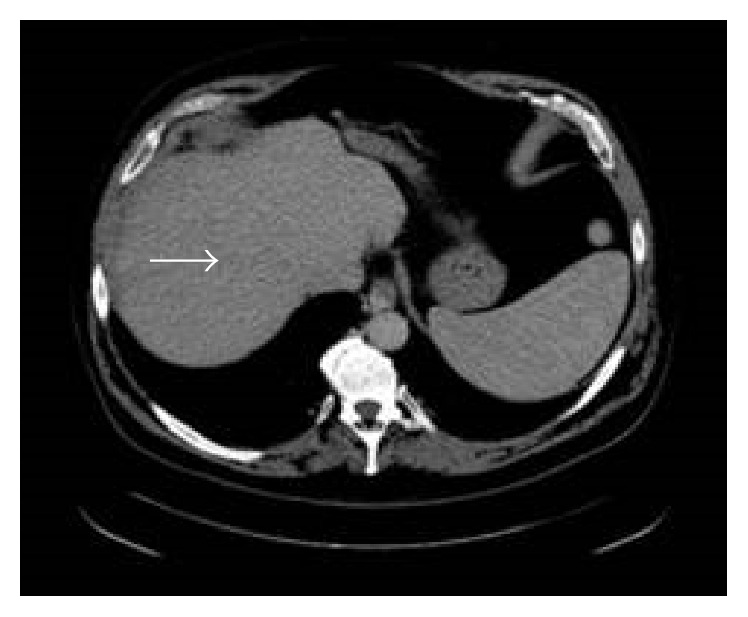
Follow-up CT image at 3 months after hepatic artery revascularization with stent placement showed resolution of liver abscess.
